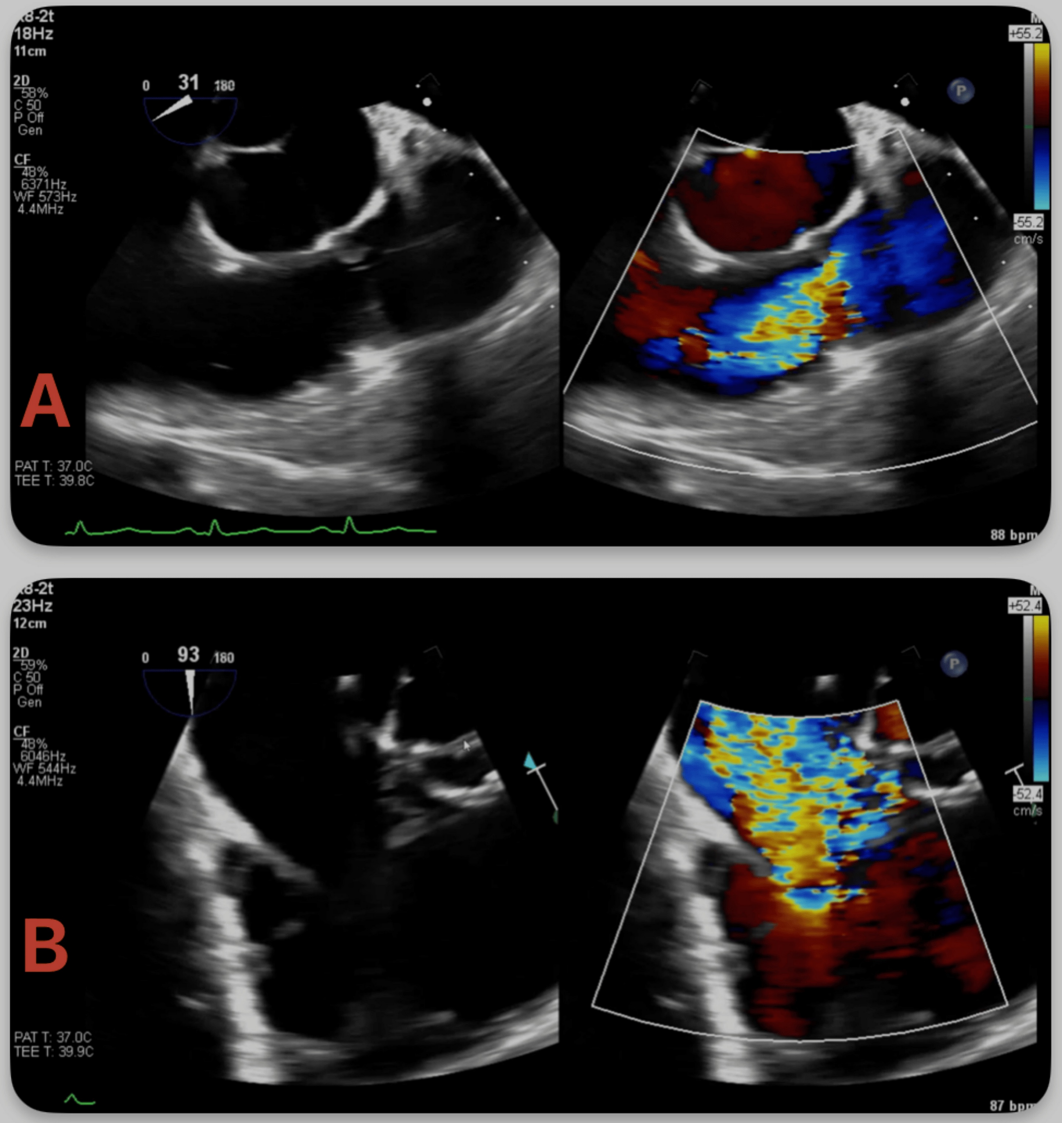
Transesophageal echocardiography (TEE) demonstrating carcinoid heart disease with dual right-sided valve involvement TEE demonstrating color compare Doppler imaging with thickened, retracted, and immobile leaflets with malcoaptation and resultant severe regurgitation of the (A) pulmonic and (B) tricuspid valves.
